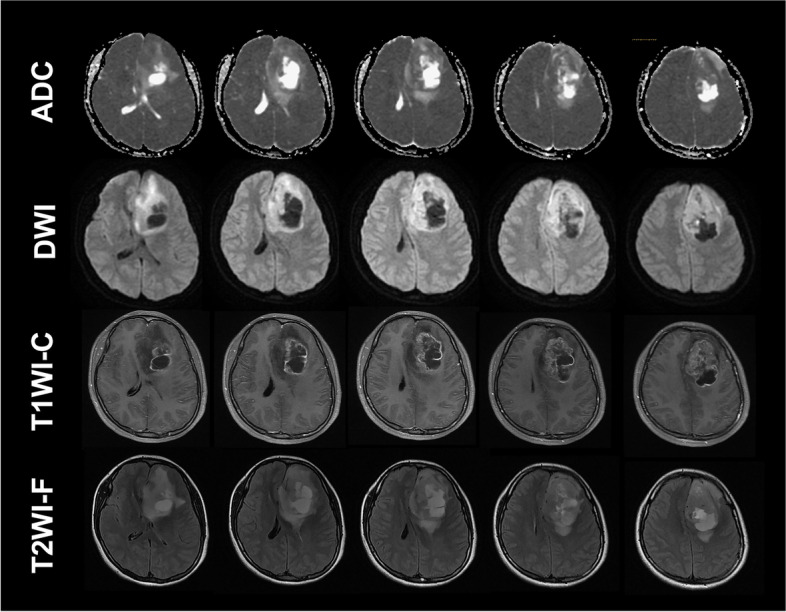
Brain MR images of the patients with grade IV glioma. Apparent diffusion coefficient (ADC), diffusion-weighted image (DWI), T1WI-contrast, and T2WI-flair images of the brain showed the occupation of the tumor and severe brain edema around the glioma was shown on the images
